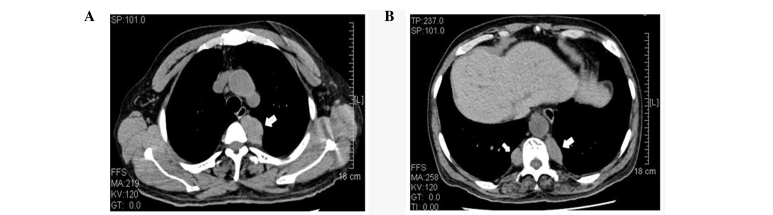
Extramedullary hematopoiesis appears as a solid homogeneous mass (green arrow) in the posterior mediastinal on a thoracic computed tomography scan. (A) T3-4 vertebral level. (B) T10 vertebral level.
